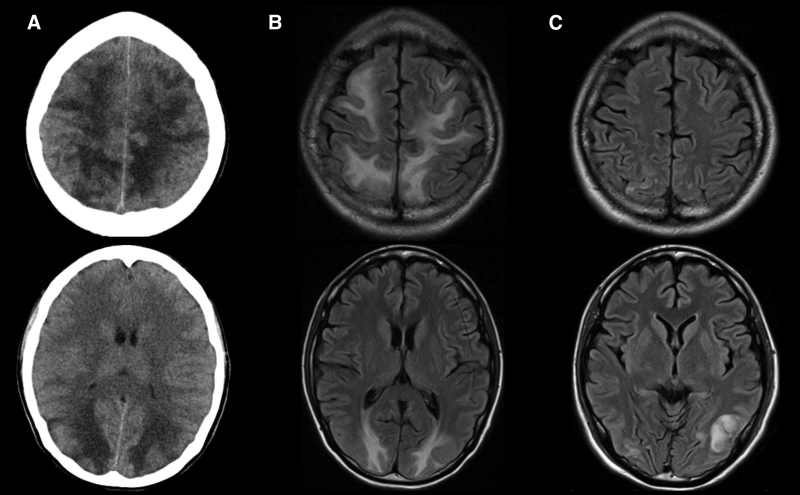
Serial neuroimaging findings during the clinical course. (A) Head computed tomography performed on hospital day 6 demonstrated widespread hypodense lesions involving the occipital and parietal lobes. (B) Brain magnetic resonance imaging (MRI) performed on hospital day 14 revealed hyperintense lesions in the same regions on fluid-attenuated inversion recovery (FLAIR) images. (C) Follow-up brain MRI on hospital day 23 demonstrated newly developed hyperintense lesions in the left temporal lobe, distinct from the previously observed lesions.
